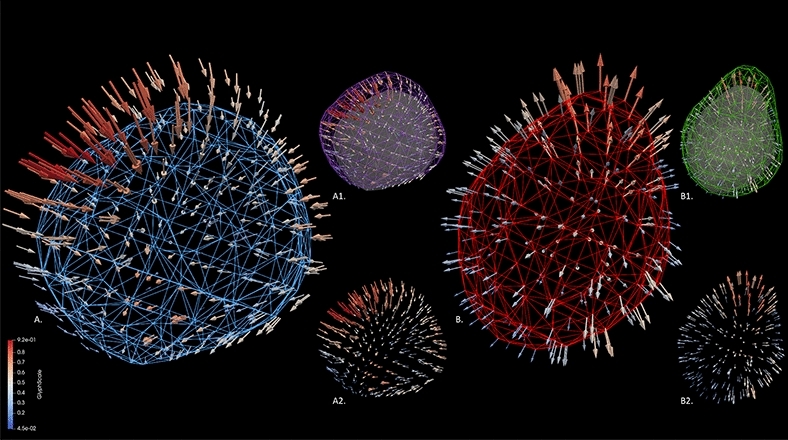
Three-dimensional (3D) displacement vectors from a (A) MS and (B) SVD lesion demonstrating both the magnitude (red indicating a higher degree of displacement) and direction (positioning of arrows) of the displacement. Blue and red meshes represent the lesion morphology at MRI time points 2 and 1, respectively. Note the asymmetric displacement inward from time point 1 (A1, purple mesh) as indicated by mixed directional vectors along with greater differences in the magnitude of change, leading to the resulting 3D shape at time point 2 for the MS lesion (A1, grey, A2). Compare to the SVD lesion with more uniform directional vectors outward from the original lesion acquired at time point 1 (B). B1. Green mesh demonstrating evolution in lesion size and relative preservation of the original shape at MRI time point 2. B2. Displacement vectors from the SVD lesion. Note the more uniform degree of change in magnitude and direction as compared to the MS lesion.
